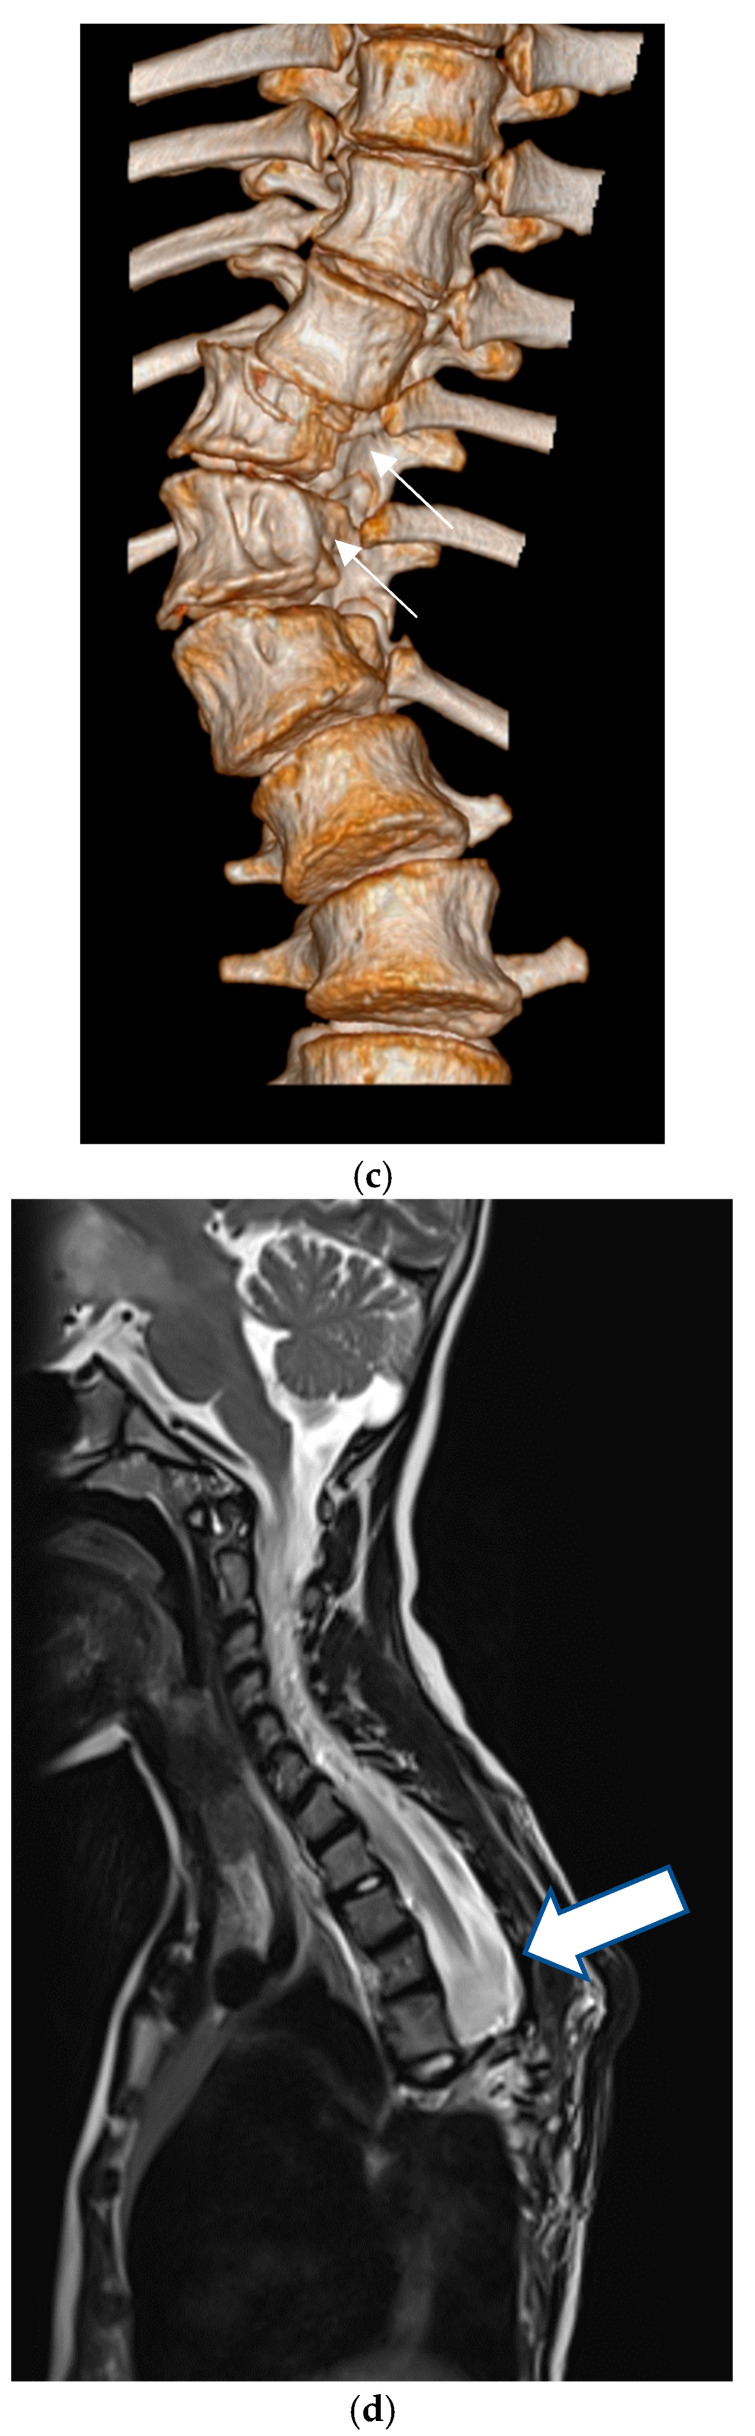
Dystrophic osseous features: (a) scalloping (thin arrow), rotation and dislocation with pathologic kyphosis (thick arrow), (b) rib penciling (white arrows), (c) wedging and rotatory dislocation (arrows) in a 3-D CT reconstruction, (d) widening of the spinal canal and dural ectasia (thick white arrow).
